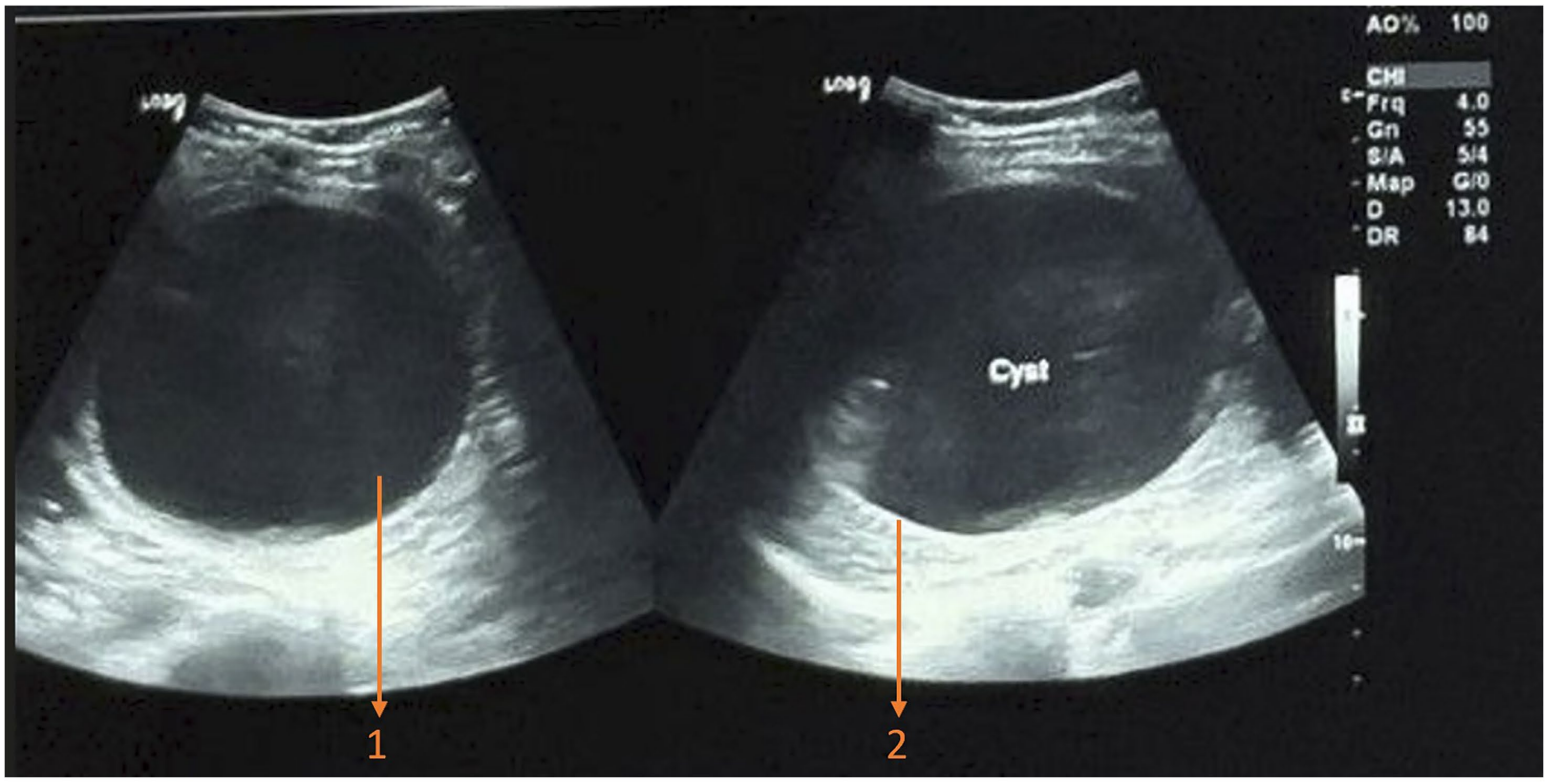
Ultrasound of the whole abdomen showing a solitary anechoic cystic lesion in the spleen. (1) Anechoic cystic cavity with no internal septations. (2) Thickened cyst wall.
